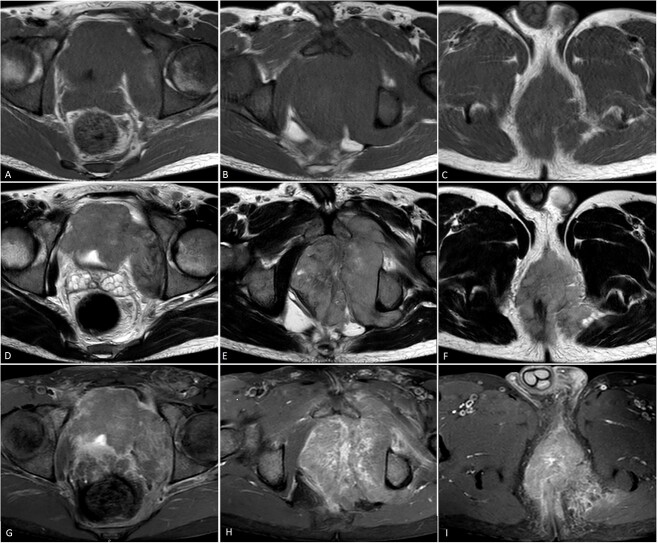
Pelvic MRI for the patient showing a huge pelvic mass, extending through the left obturator canal invading the left obturator externus muscle (E and H). The mass measures up to 12 cm in its greatest axial dimension and is noted to be iso-intense on T1W images (A–C), heterogeneously hyper-intense on T2W images (D–F), and displaying heterogeneous enhancement on Fat saturated T1W images with Contrast medium (G–I). Multiple enlarged pelvic lymph nodes were seen (Not shown here).
